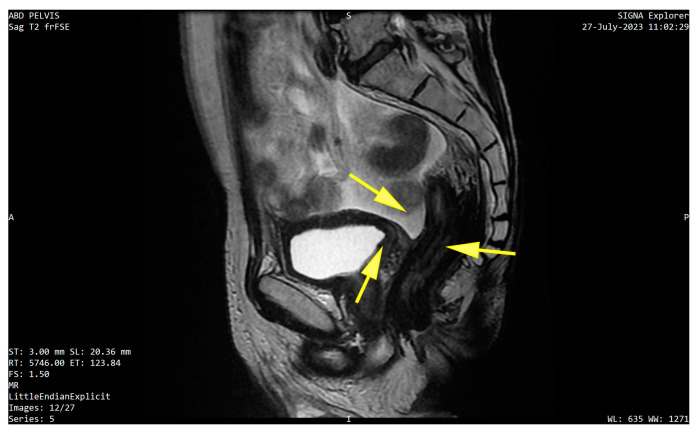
MRI sagittal images after chemoradiotherapy with arrows pointing: ascites in the rectovesical pouch and thickening of the bladder and rectal wall.
